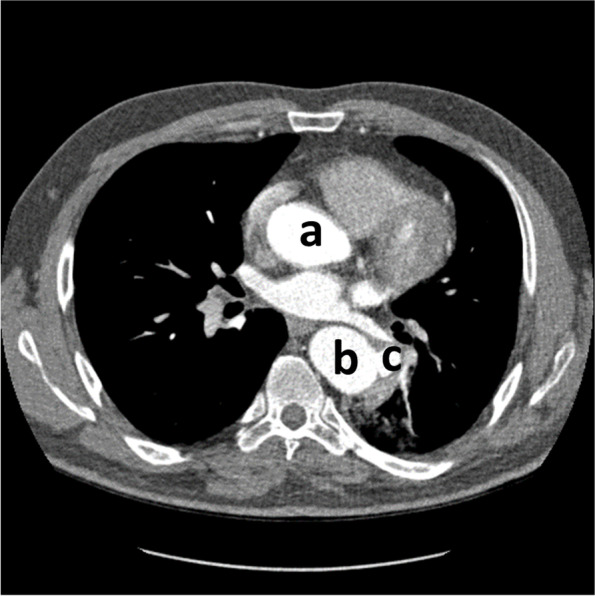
Spiral CT angiography of the Thorasic Aorta revealed two outpouchings from tube graft and fistula to bronchial artery. a: ascending aorta, b: distal anastomosis site of tube graft to descending aorta, c: outpouching from distal anastomosis site of tube graft
